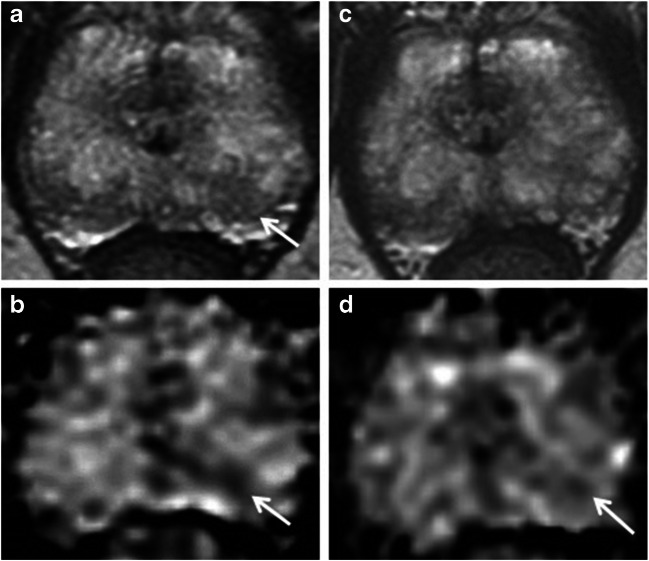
PRECISE score 2. A 70-year-old patient at enrolment, with PSA of 3.4 ng/ml. Top row: T2 axial; bottom row: ADC maps. a, b 2014 MRI shows Likert 3 lesion at the left apex PZ (arrows). Targeted biopsy shows Gleason 3 + 3 = 6 in 1/3 cores, < 5%. c, d MRI at 12 months shows more geographical features on T2 (c) and reduced conspicuity on ADC (arrow in d)
